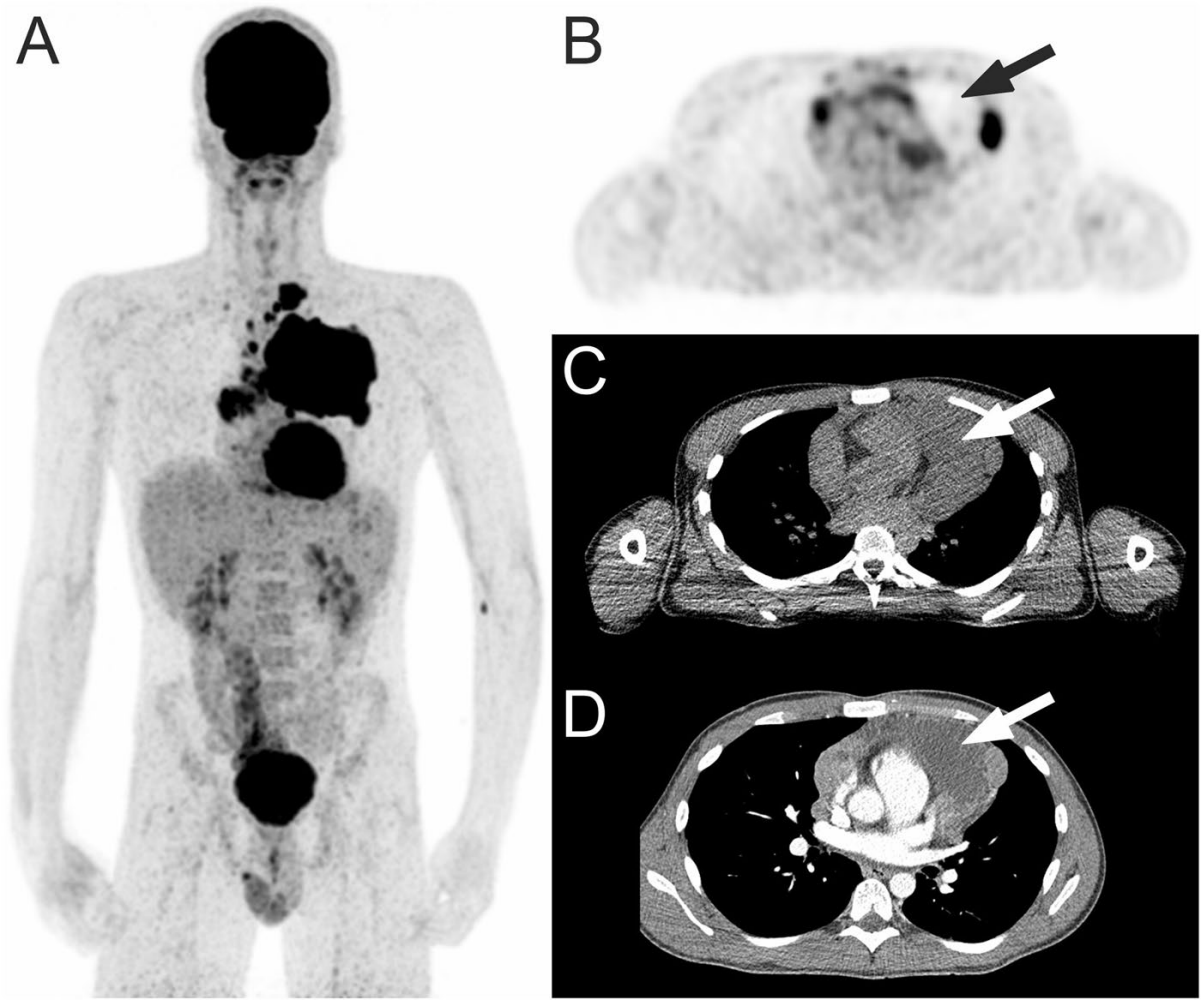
^18^F-FDG PET in a 21-year-old man with classical Hodgkin lymphoma. Coronal maximum intensity projection ^18^F-FDG PET shows a mediastinal tumor mass (A). Axial ^18^F-FDG PET with concomitant low-dose CT shows necrosis^visual^ with a photopenic area in the mediastinal tumor (B,C, arrows), with attenuation of around 18 HU on contrast-enhanced CT (D, arrow).
